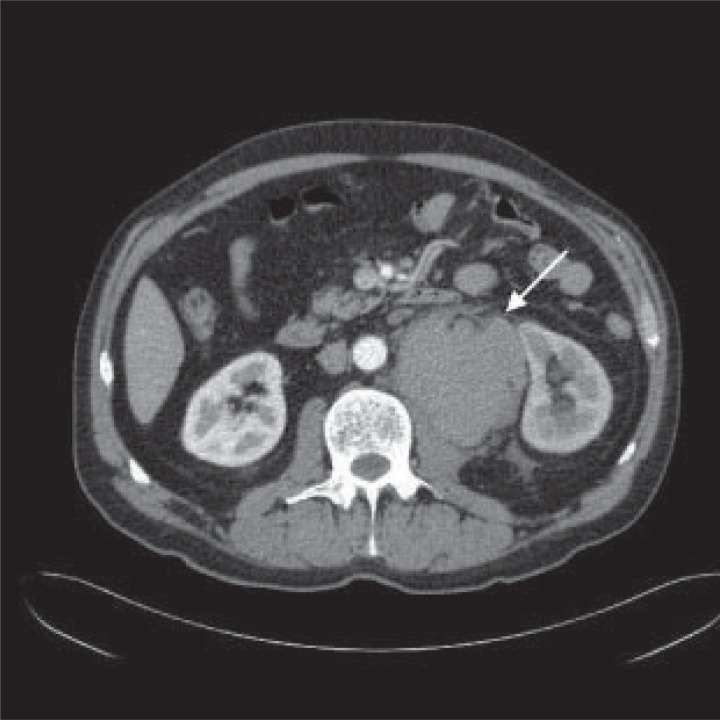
CT scan shows retroperitoneal haematoma (arrow) between left psoas muscle and left kidney.
